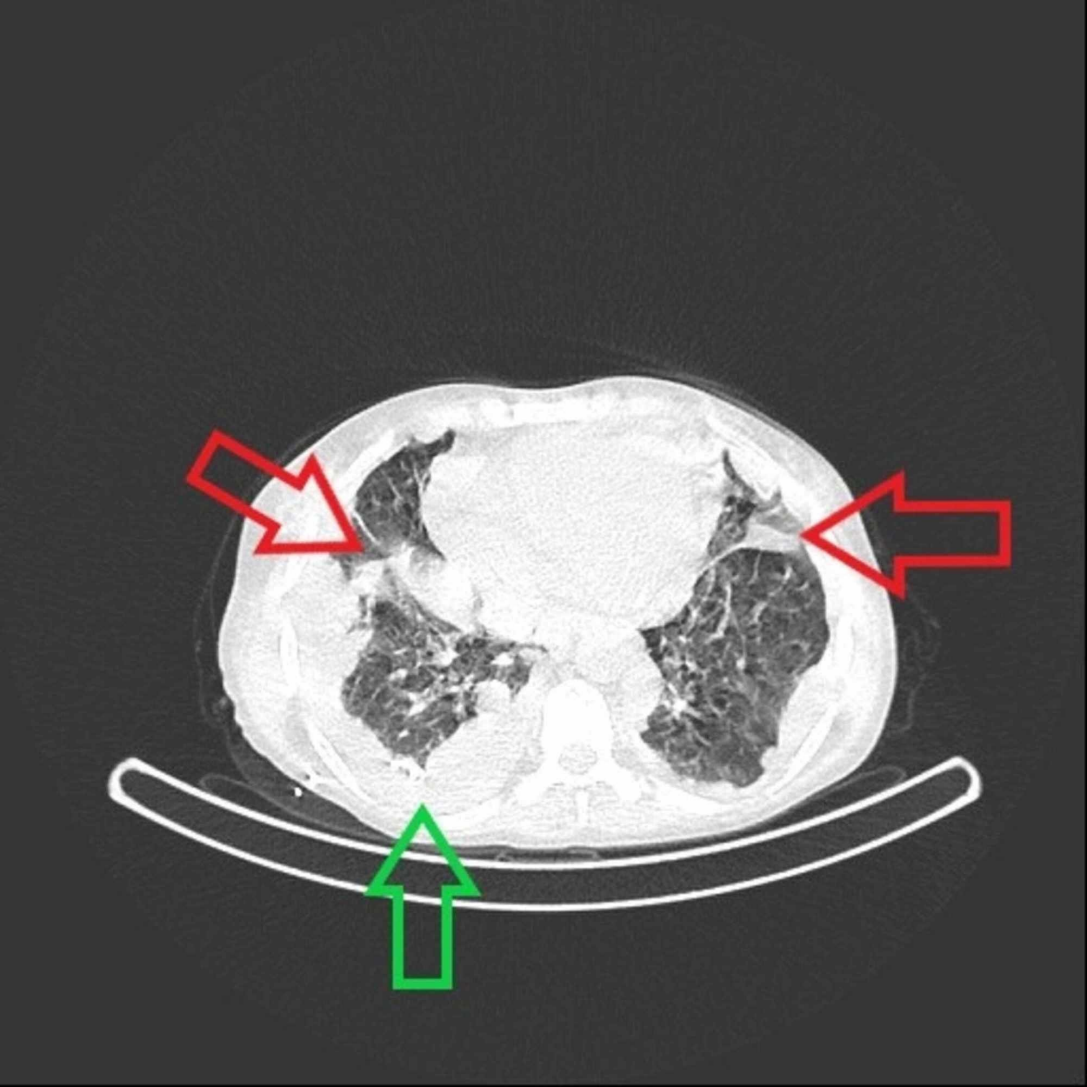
Non-contrast chest CT scan shows bilateral pleural effusion, which is more clear on the right side (green arrow), and bilateral lung consolidations (red arrows) at six months postoperatively. CT, computed tomography
